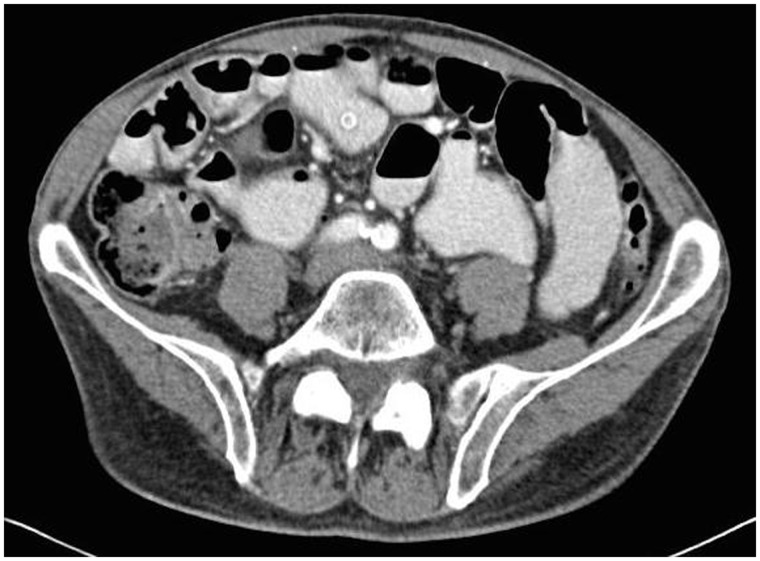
Axial CT of the abdomen and pelvis revealing what appears to be the retained capsule from a prior capsule endoscopy in the small intestine. Diffuse distension of the bowel is also present.
